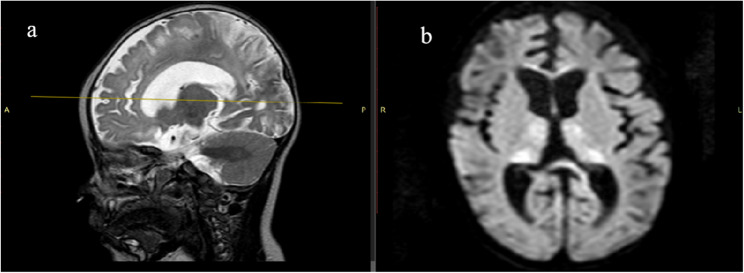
a Atrophy with multiple patchy T2 hyperintensities was observed in the cerebral hemisphere cortex. b DWI revealed abnormal signals involving the bilateral basal ganglia, thalamus, and corpus callosum
